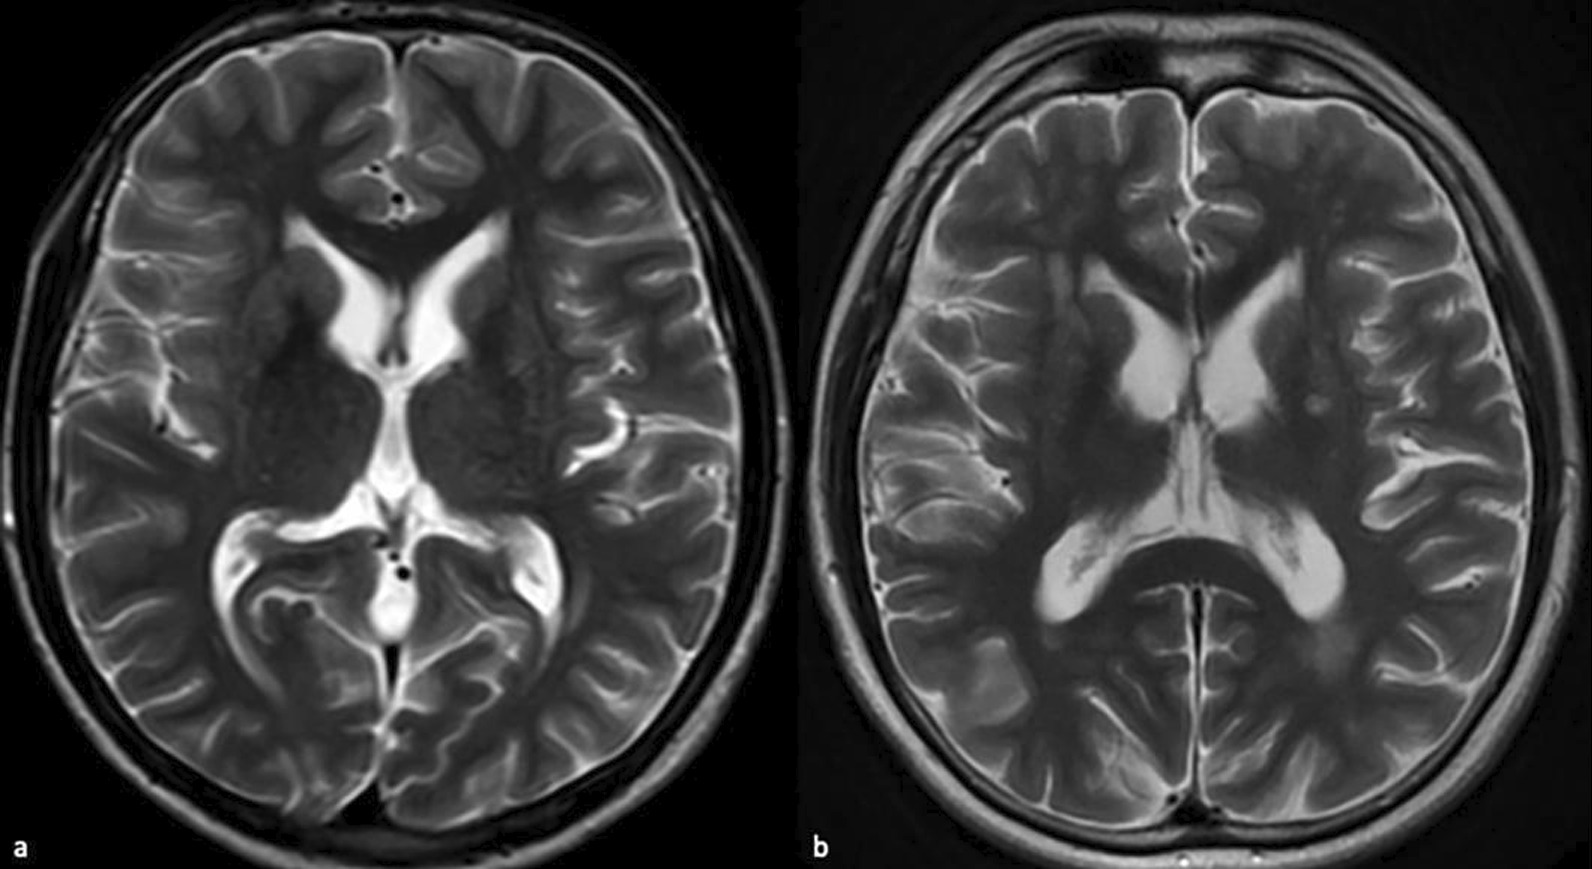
MRIs of the case in 2018 (a) and 2022 (b). Axial T2-weighted images show progression in periventricular confluent hyperintensities and cerebral cortical atrophy. Basal ganglia hyperintensities appear in 2022
